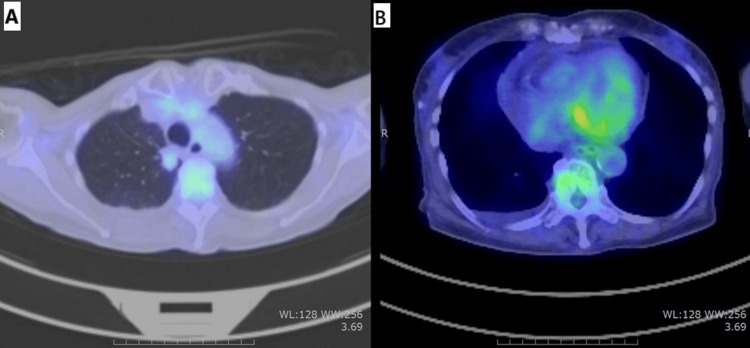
18F-FDG-PET/CT image at the time of pericardial effusion (on November 2024) The primary lesion and distant metastatic lesion also maintained a shrinking trend, and FDG accumulation was poor, so it was judged that there was no viable lung cancer lesion. (A) Primary lesion in the left upper lobe. (B) Pericardial area ^18^F-FDG-PET/CT: ^18^F-2-fluoro-2-deoxy-D-glucose positron-emission tomography/computed tomography
